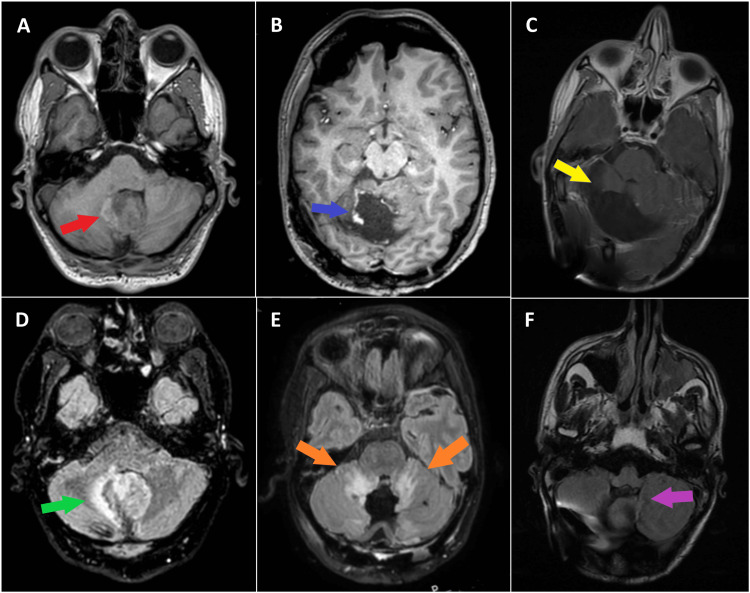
Serial MRIs of a Young Male With Recurrent High-Grade Glioneuronal Tumor Treated With Larotrectinib The top row depicts serial gadolinium-enhanced T1-weighted MRI images at recurrence with a posterior fossa mass with areas of enhancement (red arrow) (A), postoperatively with residual enhancement at the resection cavity (blue arrow) (B), and after six months of larotrectinib treatment with resolution of enhancement (yellow arrow) (C). The bottom row depicts serial, T2-weighted, fluid-attenuated inversion recovery (FLAIR) MRI images at recurrence with a T2 hyperintense mass and surrounding vasogenic edema (green arrow) (D), postoperatively with persistent vasogenic edema surrounding the resection cavity (orange arrows) (E), and after six months of larotrectinib treatment with resolution of the abnormal T2 signal (purple arrow) (F).
